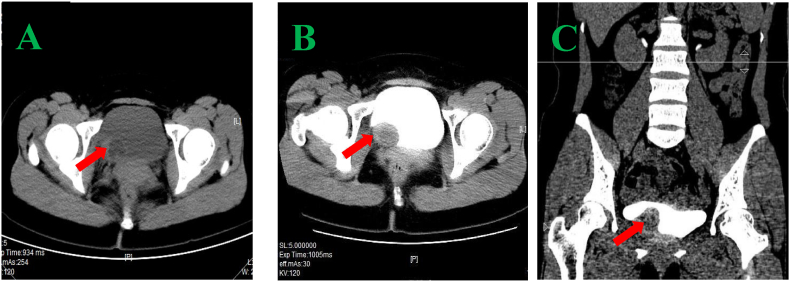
CT image (A–C): A pelvic cystic-solid nodular shadow (33 mm × 27 mm) with a clear boundary. The enhancement scan shows noticeable enhancement of the cystic wall and wall nodule, low density within the cyst, and no thickening of the adjacent bladder wall.
